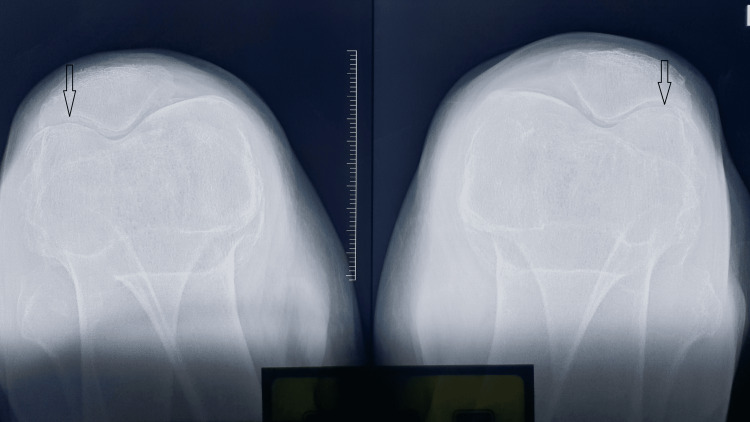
Skyline view plain radiography of both knee joints suggesting features of arthritis (patellofemoral joint space narrowing).
